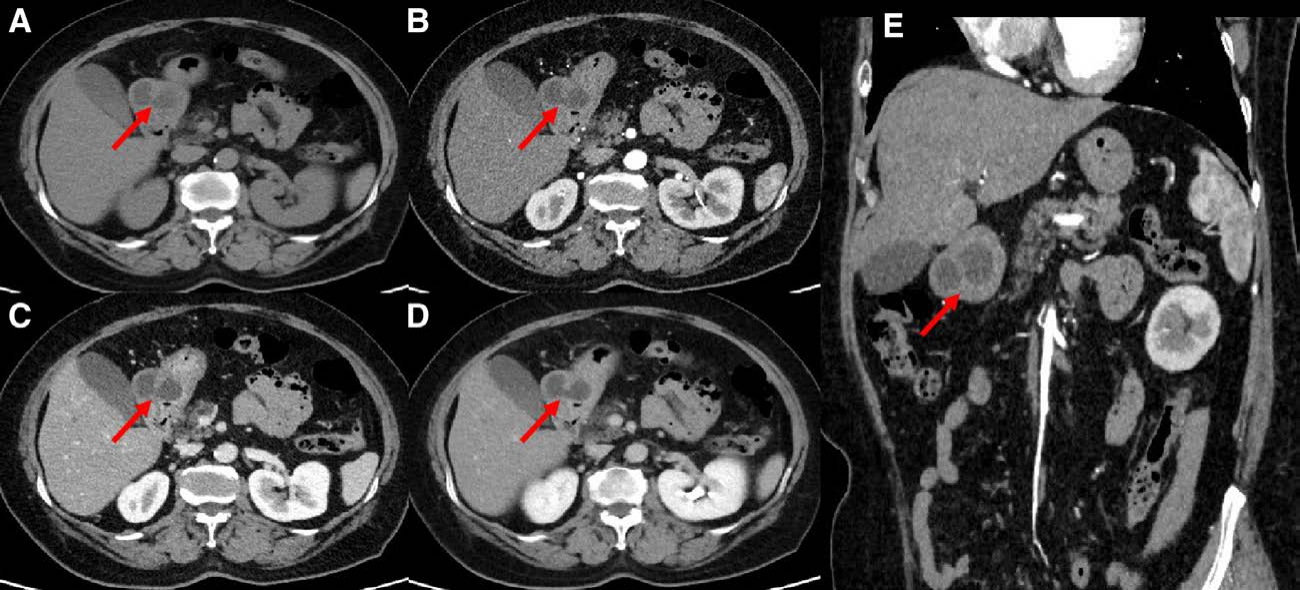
(A–E) represented axial CT plain scan, arterial phase, venous phase and delayed phase of enhancement scan, coronal arterial enhancement, respectively, showing the cystic lesion of gastric antrum with a larger axial cross-sectional area of 49 mm × 35 mm. The lesion was in the shape of ovate, with well-defined margin. The diameter of the thickest part of the cyst wall was about 9 mm, the CT values of plain and enhancement phase III were about 41HU, 45HU, 61HU and 70HU, respectively. The cyst contents showed low density and no enhancement, the CT values of plain and enhancement phase III were about 5HU, 6HU, 8HU, 9HU.
